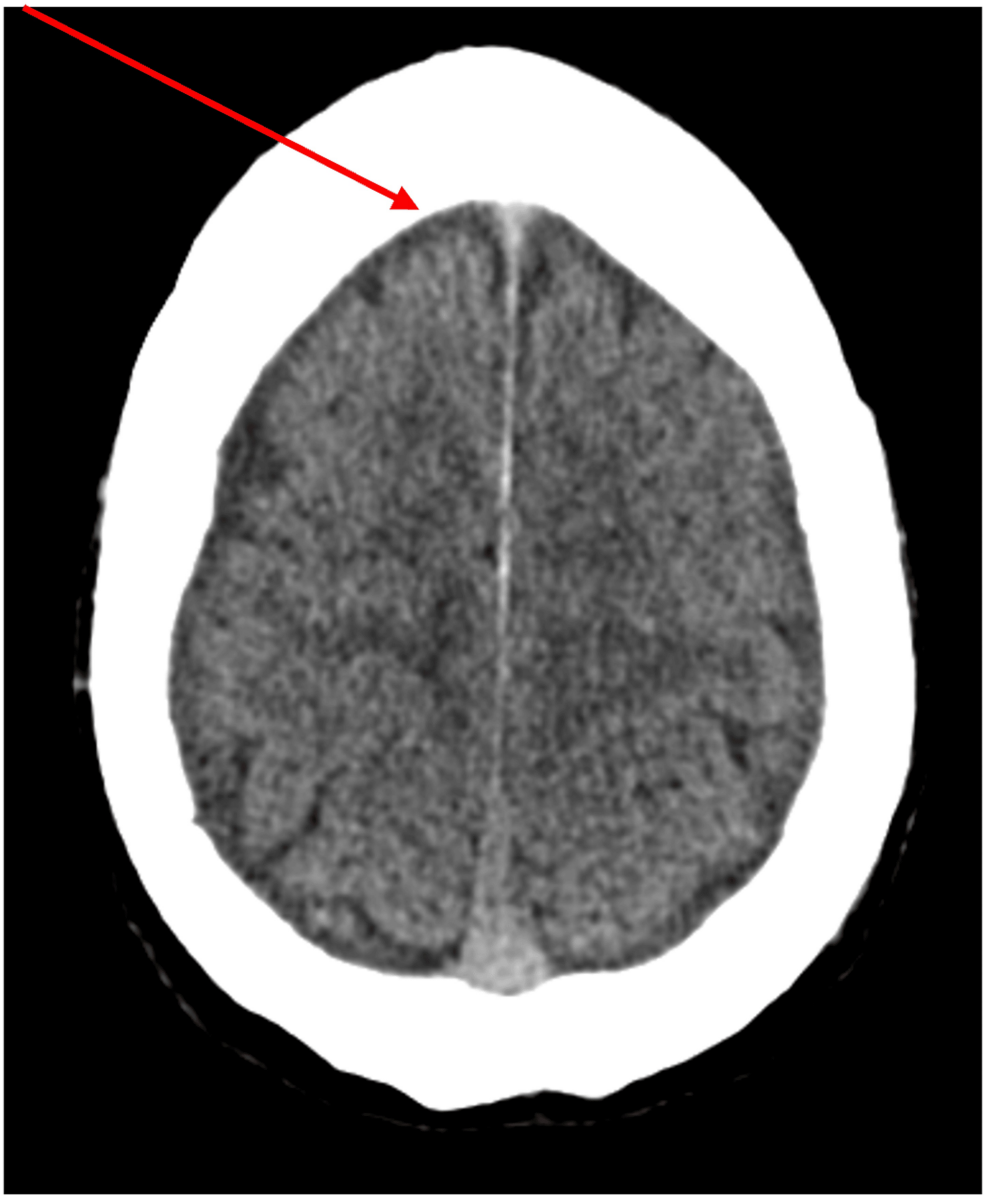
CT head showing the prominence of the subdural spaces bilaterally within the vertex overlying the frontal lobes
